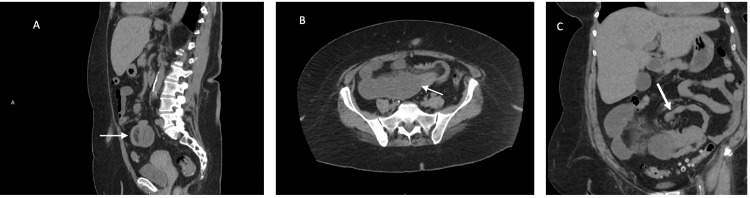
Non-contrast abdominal CT of the patient. The images show (A) sagittal view significant for an incomplete target sign, (B) transverse view of the intussusception, and (C) in the coronal view the whirl sign became evident.
